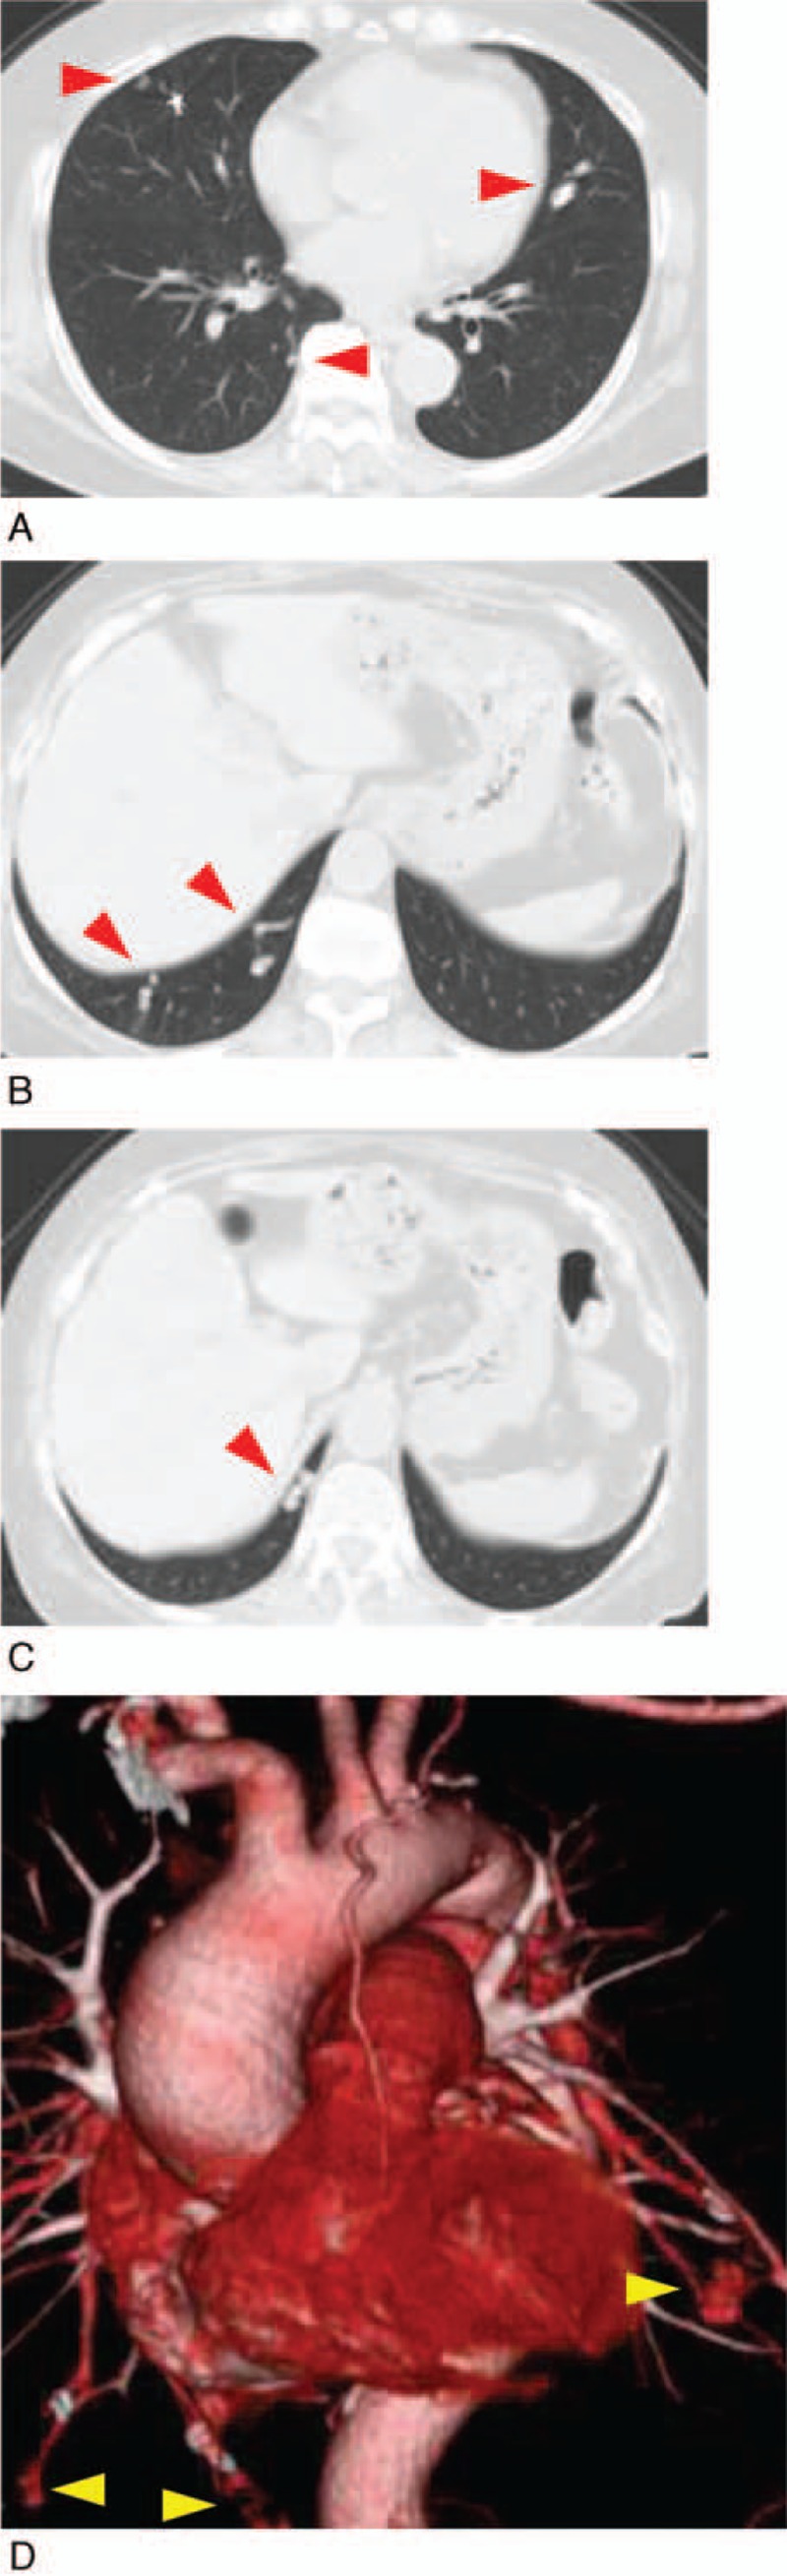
Chest computed tomography (CT) revealed multiple pulmonary arteriovenous malformations (PAVMs) in the middle lobe S4 of the right lung, lower lobe S6, S9, and S10 of the right lung, and upper lobe S4 of the left lung (A–C: red arrow heads). Three-dimensional reconstruction multidetector CT revealed multiple PAVMs (D: yellow arrow heads).
